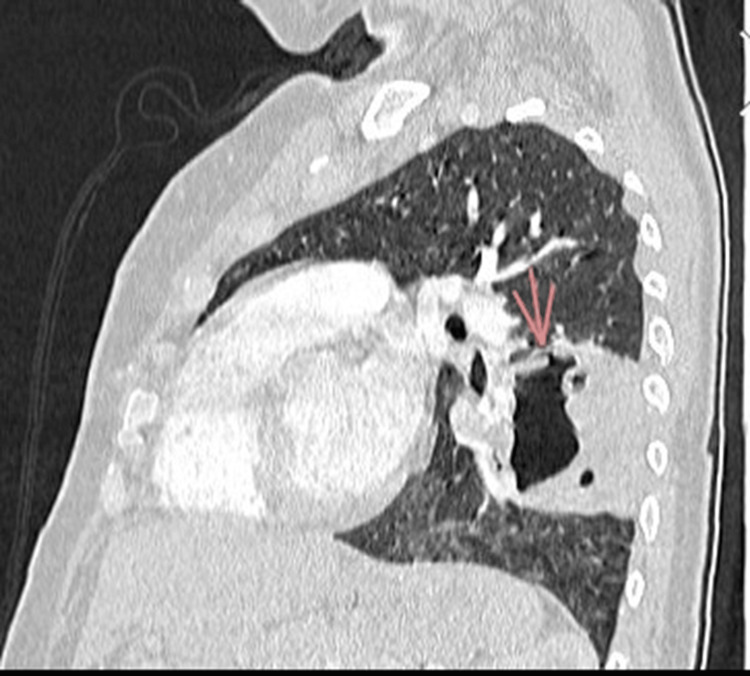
Sagittal chest CT reconstruction in lung window showing the communication between the cavity of the ruptured hydatid cyst and the bronchial tree (arrow), confirming the bronchial fistulization.
